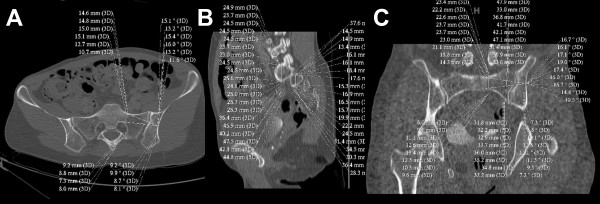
Technique and obtained CT measurements summary in the axial (panel A), sagittal (panel B), and coronal planes (panel C). See text for details and explanations.
